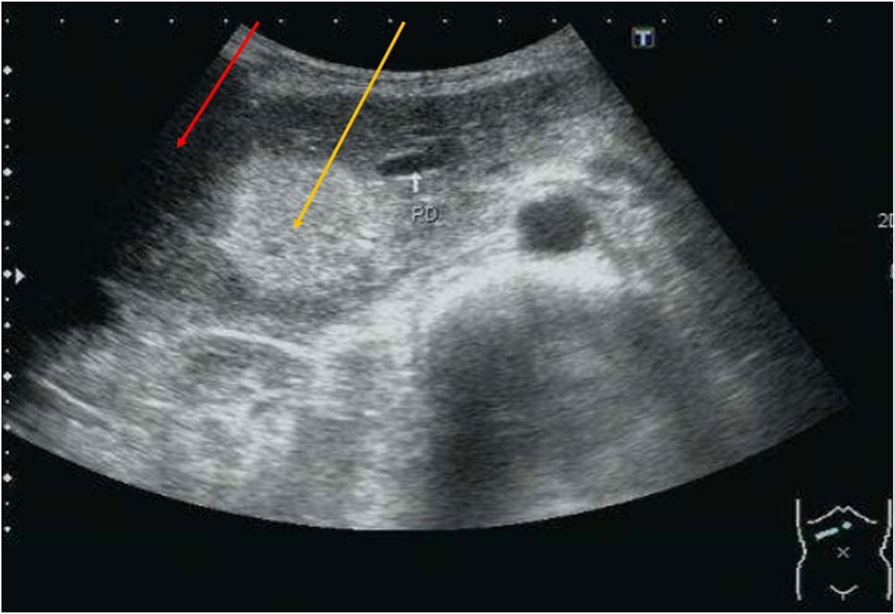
Ultrasound image. Echo-guided needle biopsies were performed at the high echoic central part (
